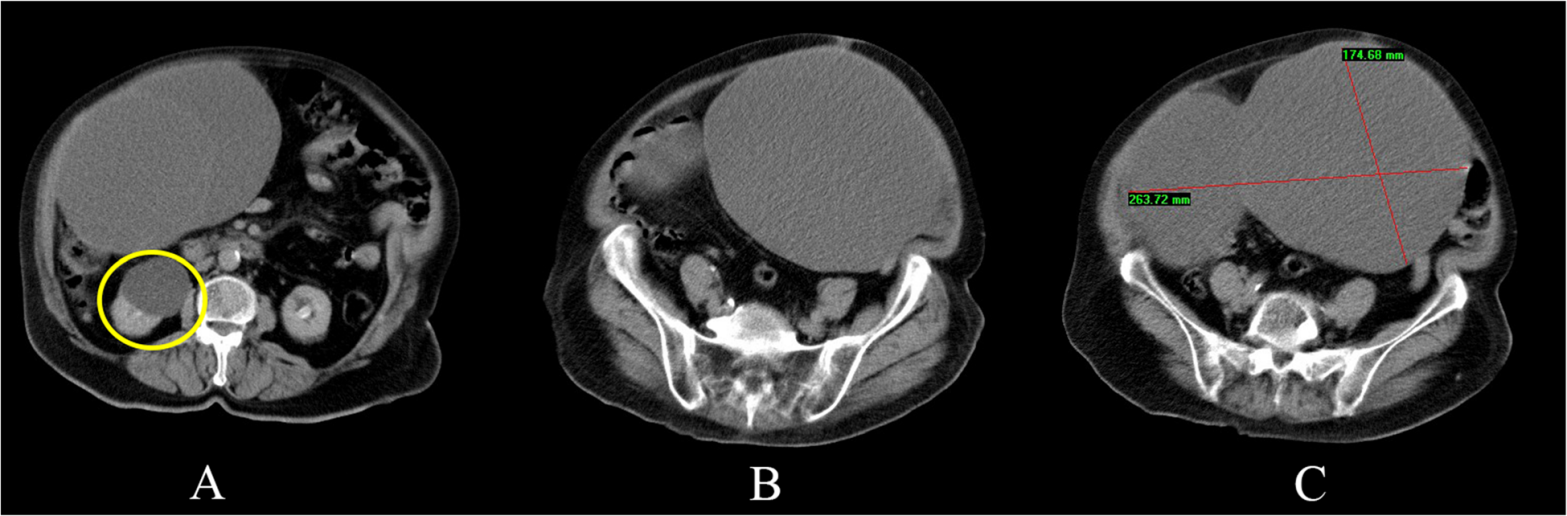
Computed tomography scan of the abdomen showing the homogenous cystic mass filled with isodense fluid. Note the presence of a right-sided hydronephrosis (yellow circle)
